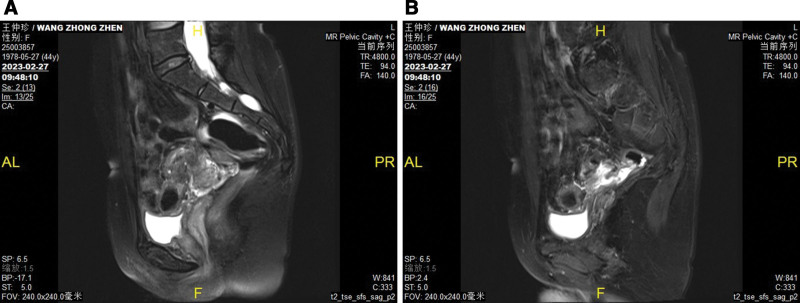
(A and B) MRI showed localized thickening of the intestinal wall in the sigmoid colon near the descending colon, and a lumpy abnormal signal shadow was seen next to the intestinal canal.
